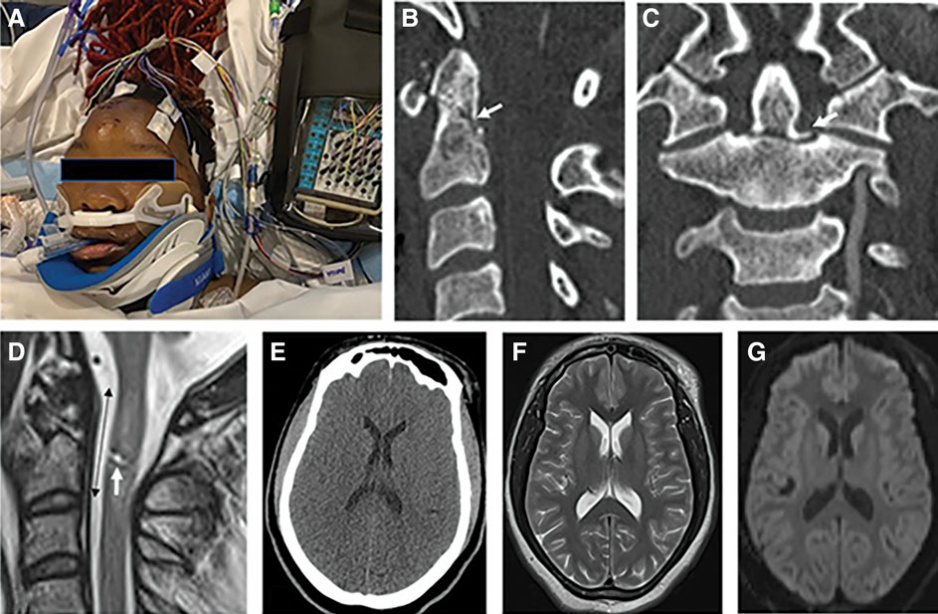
This 33-year-old woman was an unbelted rear-seat passenger during a motor vehicle accident. At the scene of the accident, the emergency medical technicians found her in complete cardiorespiratory arrest. After resuscitation, she was airlifted to the trauma center. In the trauma center, she had one more episode of cardiac arrest followed by continuous facial myoclonus (A). Her Glasgow Coma Scale score was 3; pupils were small and minimally reactive. There were no corneal reflexes, and cold calorics did not induce any horizontal movement of the eyeballs. CT scan of the cervical spine showed type II odontoid fracture with minimal displacement (B,C). T2-weighted MRI images indicated near transection of the spinal cord immediately behind the injury epicenter and spinal cord swelling. Intramedullary lesion length was 28.8 mm (D). Brain CT and MRI were negative for TBI and ischemic stroke (E,F,G), except for a focal point of infarction in the cerebellum attributable to blunt cerebrovascular injury to the right vertebral artery (C). Diffusion tensor images did not reveal any evidence of restricted diffusion in the basal ganglia or cortex (G). CT, computed tomography; MRI, magnetic resonance imaging; TBI, traumatic brain injury.
